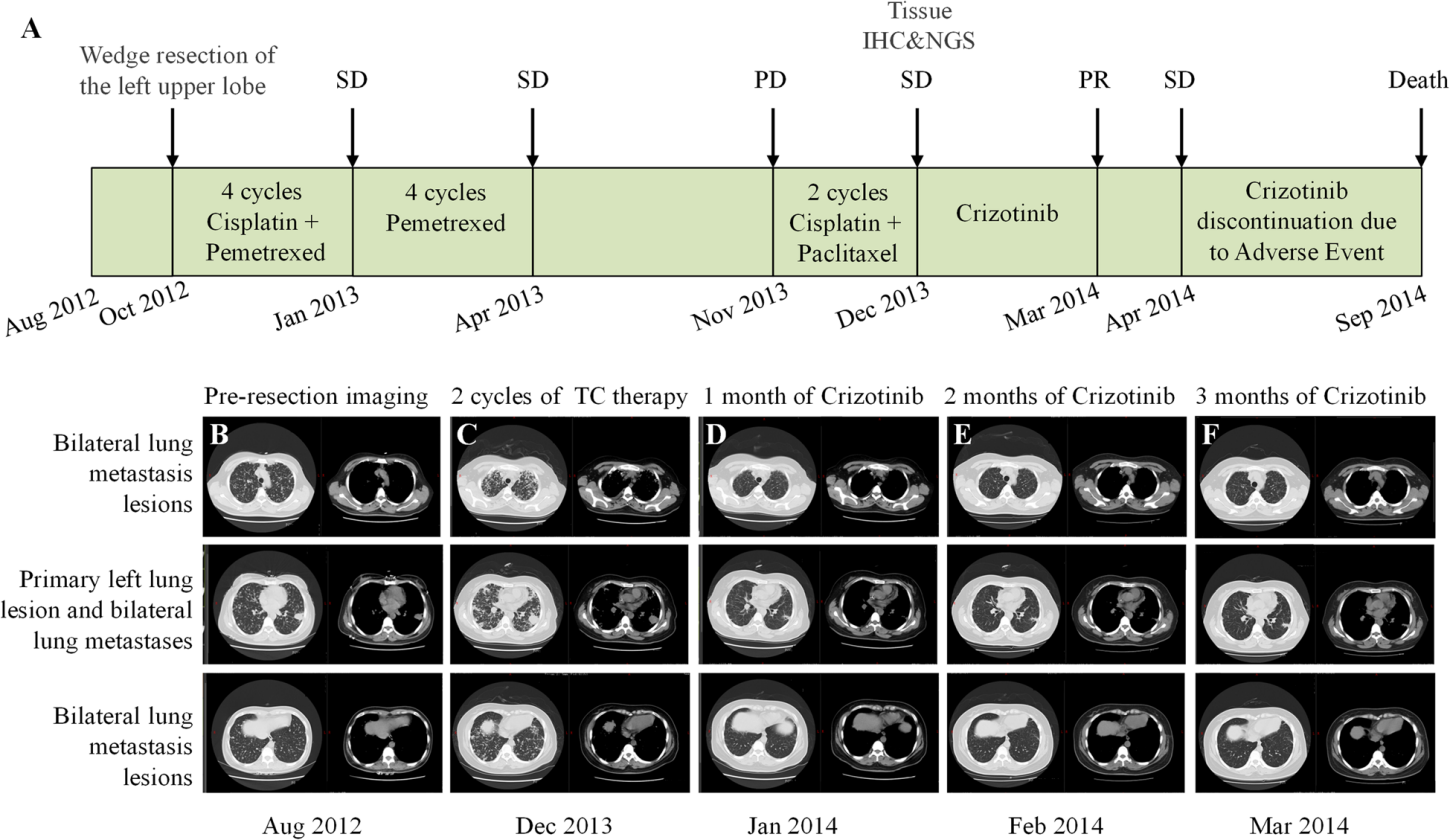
Timeline of the case 1’s disease from diagnosis to death (A). Chest computed tomography (CT) scans at diagnosis (B), and after 2 cycles of Cisplatin + Paclitaxel (C), subsequent chest CT scans performed 1 month (D), 2 months (E) and 3 months (F) after initiation of crizotinib treatment
